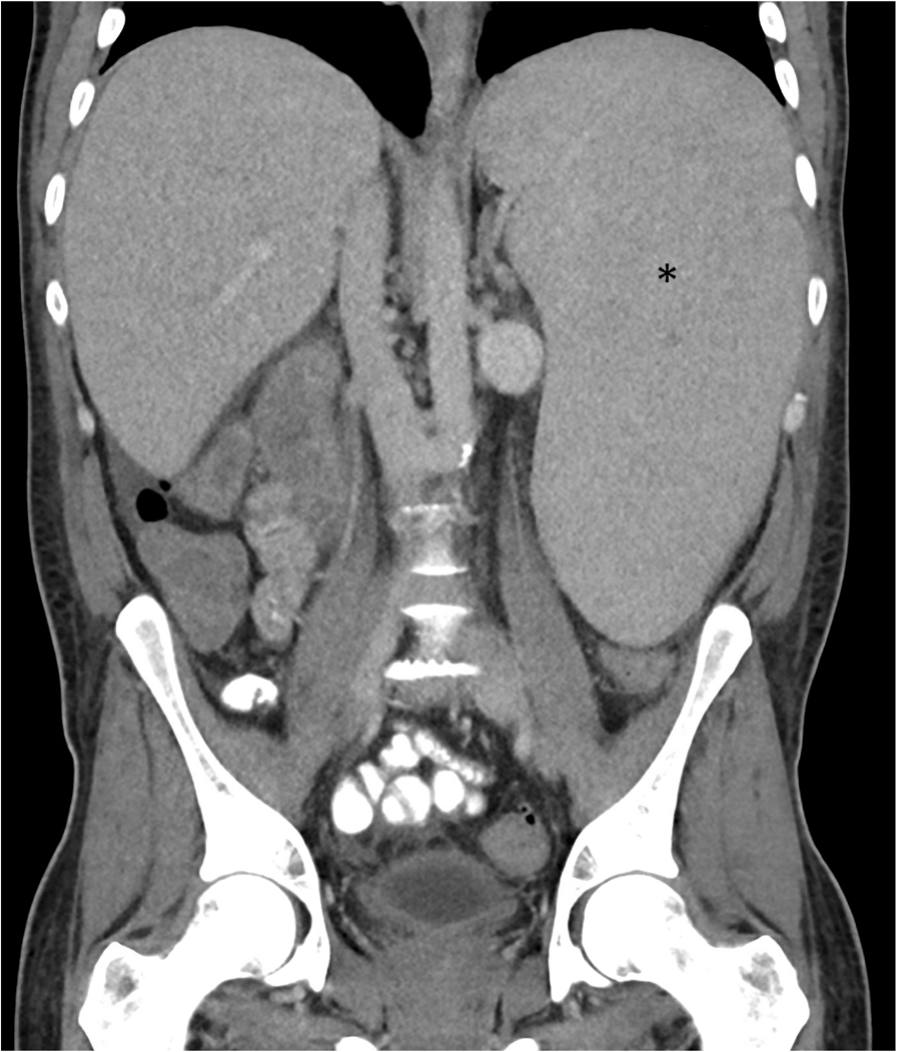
Coronal CT of the abdomen demonstrating a markedly enlarged spleen (asterisk). The combination of osteosclerosis and massive splenomegaly has a narrow differential diagnosis and is most suggestive of myelofibrosis
